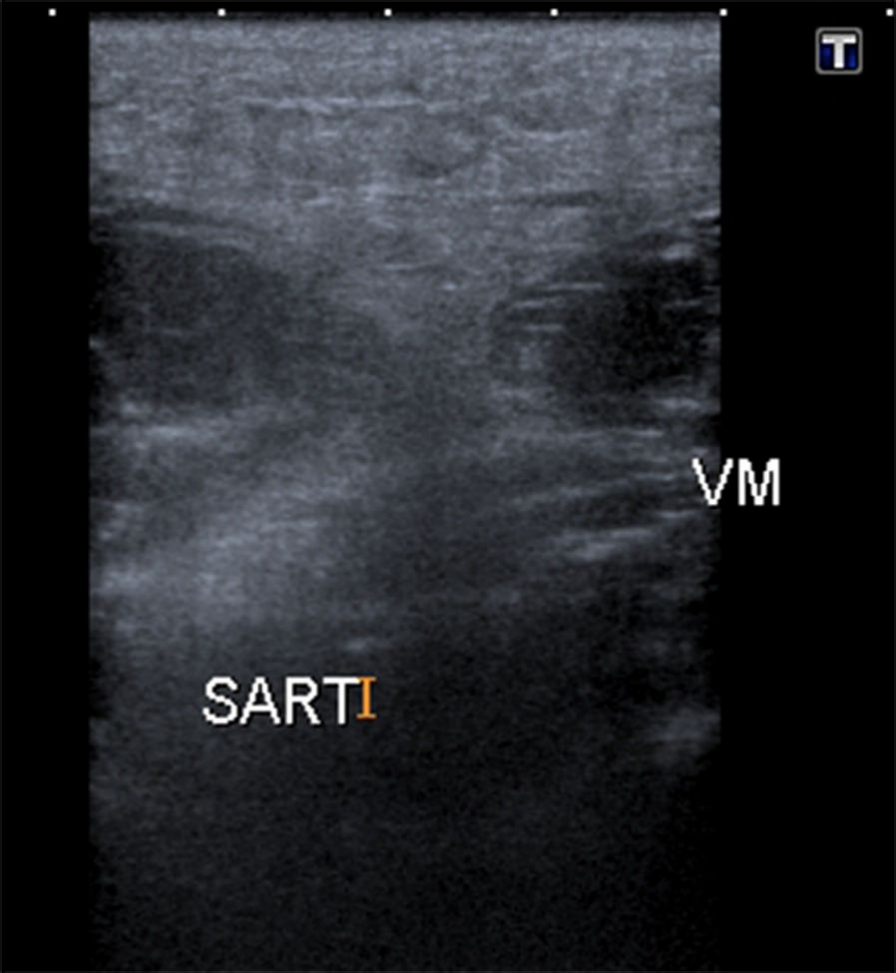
Transverse ultrasound image of sartorius muscle
